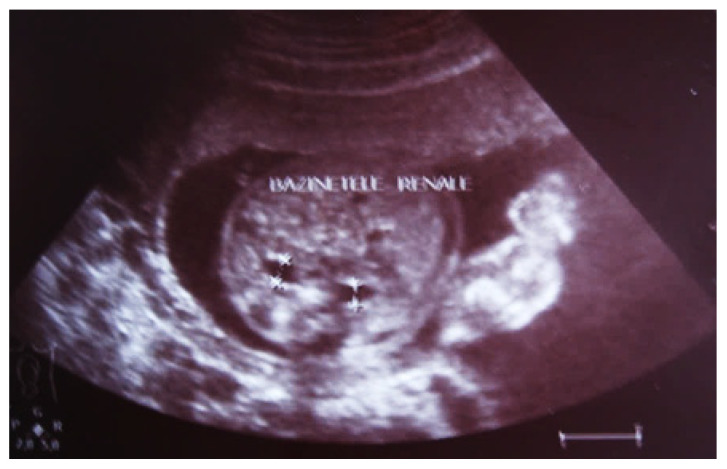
Intrauterine ultrasound at the 21st week of pregnancy. Ultrasonography—the fetus corresponds to the age of 20–21 weeks. Bilateral renal pyelectasia.
